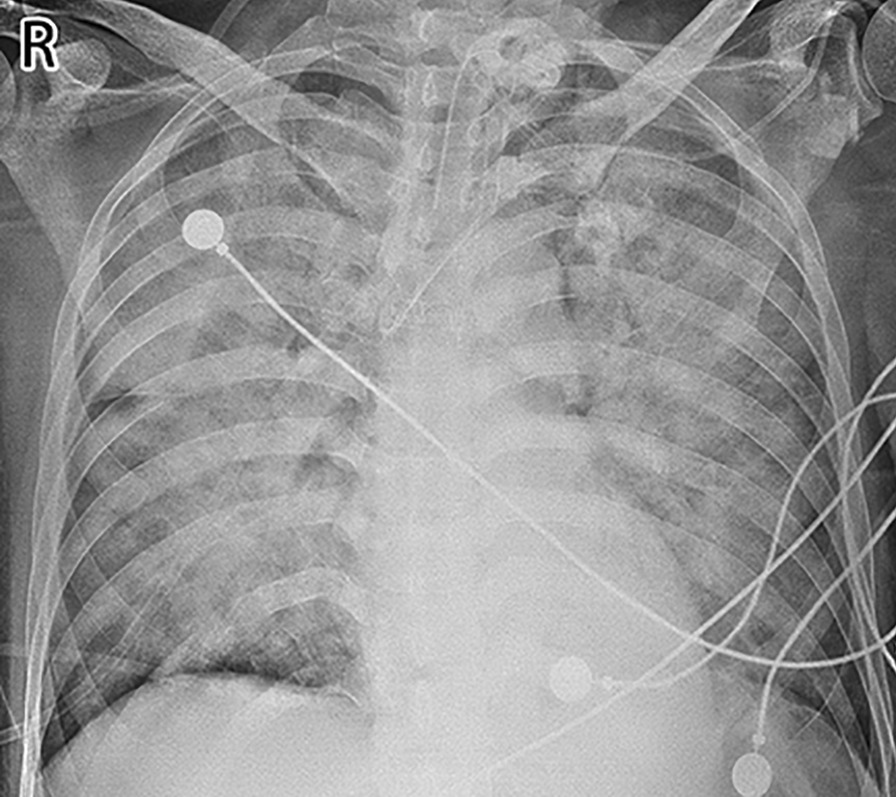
The bedside chest radiograph after surgery. The X-ray found bilateral diffuse opacities in the lung after surgery
